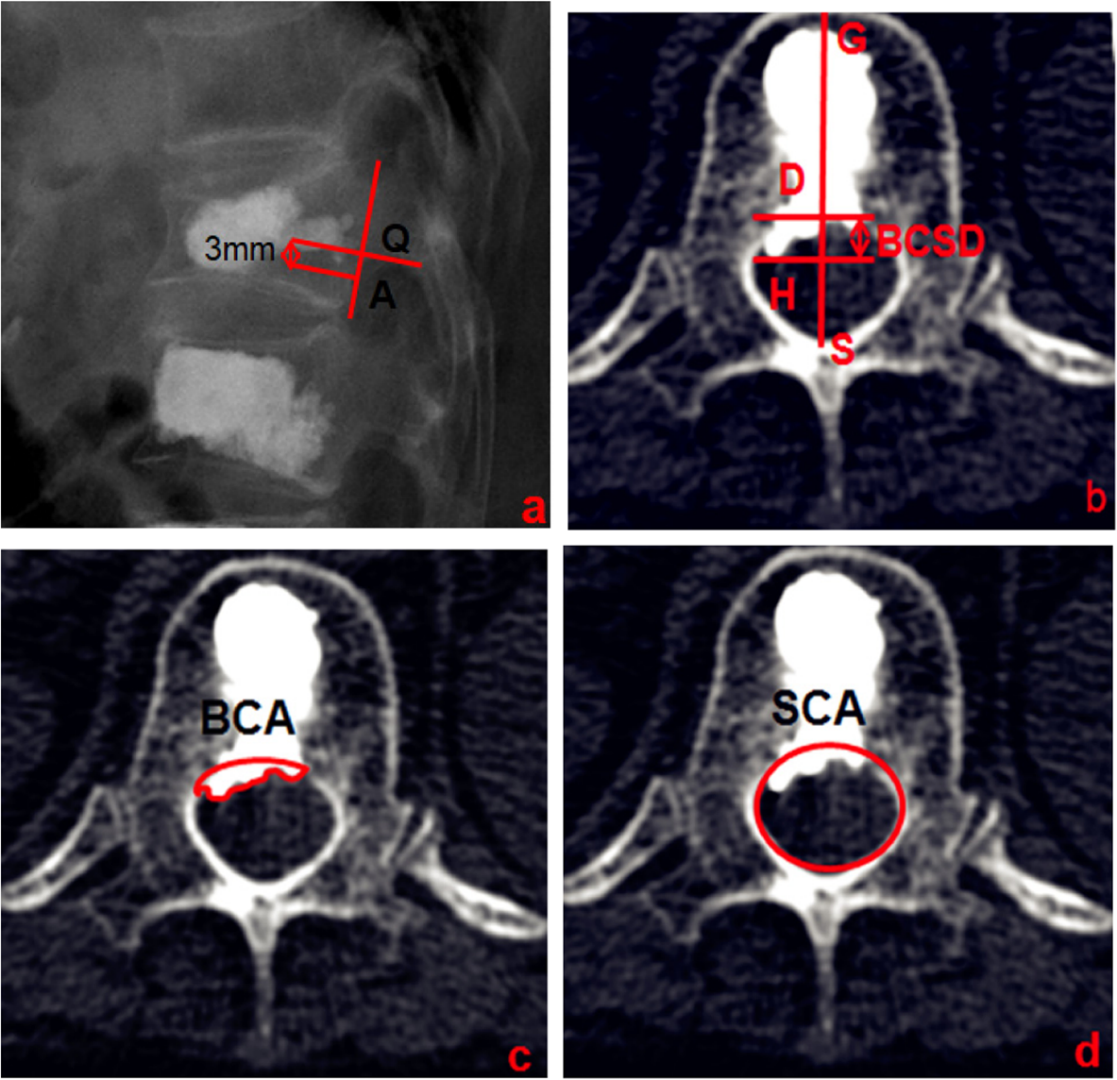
a Bone cement distribution near the L2 line. b CT transverse image of the vertebral body in figure a suggests bone cement leakage into the spinal canal. BCSD represents the maximum sagittal diameter of bone cement leakage into the spinal canal. c BCA shows the area of bone cement leakage into the spinal canal. d SCA shows the area of the bony spinal canal at the same level as b
